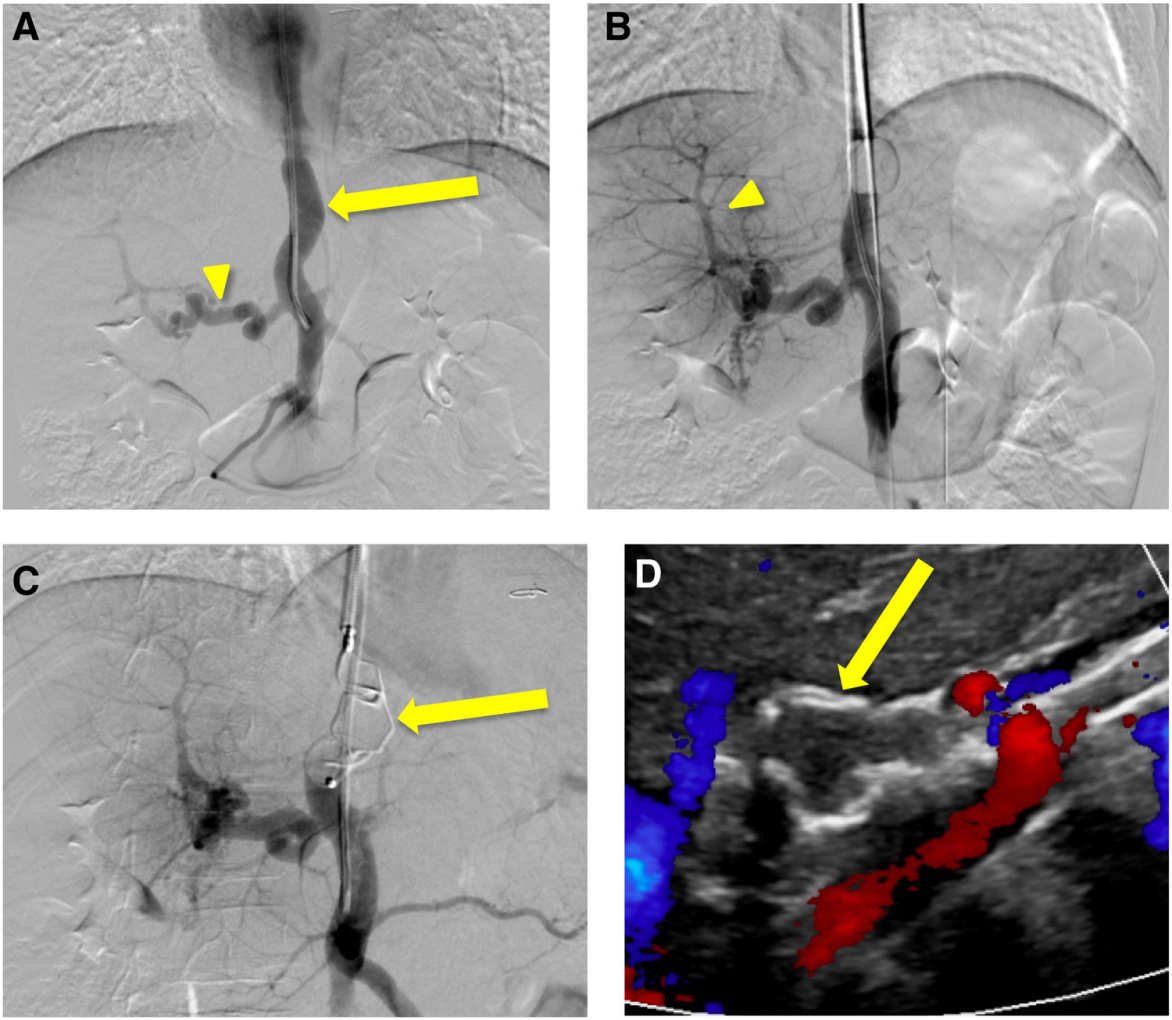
Interventional Radiology test occlusion venograms, embolization and follow-up imaging of the portosystemic shunt. A) Venograms demonstrated cavernous transformation of the main portal vein (arrowhead) and a long and narrow intrahepatic portosystemic shunt (arrow) connecting the superior mesenteric vein/splenic vein confluence with the left hepatic vein. B) Occlusion venogram showed multiple intrahepatic portal vein radicals (arrowhead). C) A 16-mm Amplatzer 2 plug (arrow) was deployed at the proximal aspect of the shunt. D) Follow-up ultrasound imaging shows complete occlusion of the shunt with the Amplatzer 2 plug (arrow) and preservation of flow within the left hepatic vein and left portal vein.
